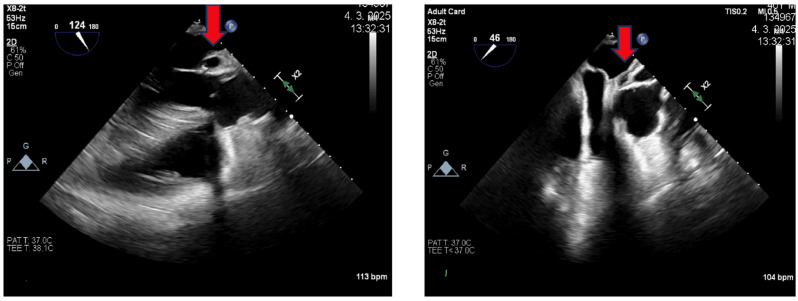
Transesophageal echocardiography: Long and short axis projection of the aorta. The red arrow shows a pseudoaneurysm.
